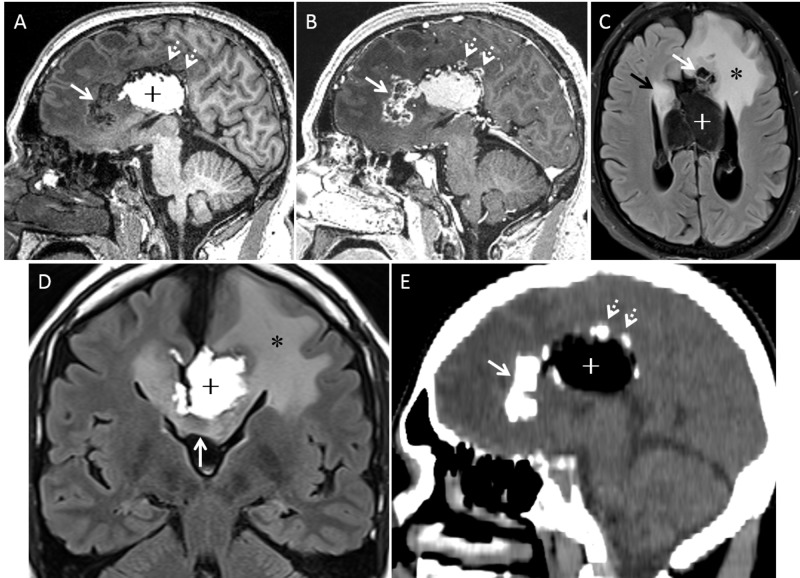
Preoperative images. A) Noncontrast left parasagittal 3D T1 image demonstrates nodular calcified lesion in the left anterior medial frontal lobe extending towards the cingulate gyrus (solid arrow). Small nodular calcifications (dotted arrows) at the junction of left cingulate gyrus and the pericallosal tubulonodular lipoma (+). B) Post contrast left parasagittal 3D T1 image shows peripheral enhancement of the nodular calcified lesions (solid and dotted arrows). C) Axial fat saturated FLAIR image demonstrates the nodular calcified lesion in the medial frontal lobe and cingulate gyrus (solid white arrow). Note the vasogenic edema involving much of the left frontal lobe (*). Lipoma appears dark from fat saturation (+). Focal edema in the right cingulate gyrus (black arrow). D) Coronal FLAIR image shows the lipoma (+) mainly located on the left side in the interhemispheric fissure. Genu of the corpus callosum shows extension of edema towards the right cingulate gyrus (arrow). Note the vasogenic edema left frontal lobe (*). E) Sagittal reformation of noncontrast CT demonstrates the nodular foci of calcifications (solid and dotted arrows) along with the hypoattenuating lipoma (+). CT: Computed tomography; FLAIR: Fluid-attenuated inversion recovery.
